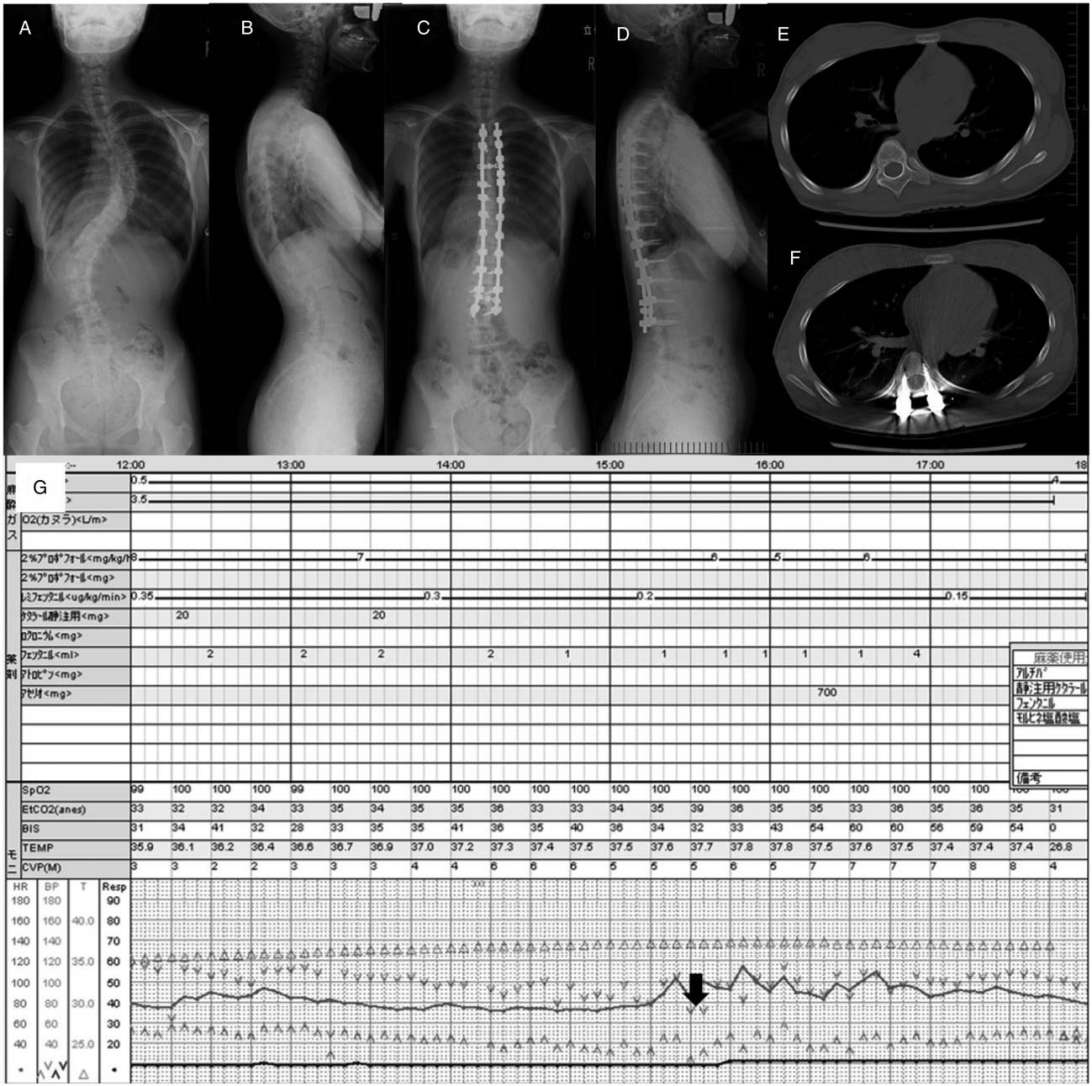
Case with hypotension alarm during surgery. A and B were the radiographs before surgery; C and D were after surgery. E and F were axial CT at the level of the apical vertebra before and after surgery. G was the record of the anesthesia and arterial pressure decreased at DVR procedure, shown by the black arrow. CT = computed tomography, DVR = direct vertebral rotation.
